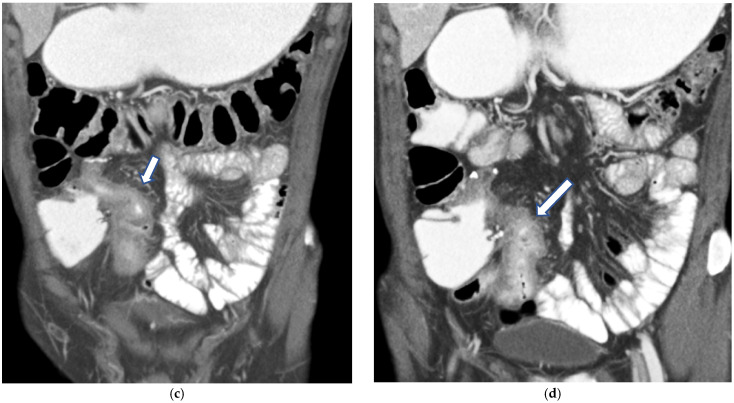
(a,b) Axial CT scan in the venous phase after administration of positive oral contrast medium (Gastrografin) showing thickened terminal ileum in a 65-year-old patient with Crohn’s disease. (c,d) Coronal CT scan in the venous phase after administration of positive oral contrast medium (Gastrografin) showing thickened terminal ileum (arrows) in a 65-year-old patient with Crohn’s disease.
